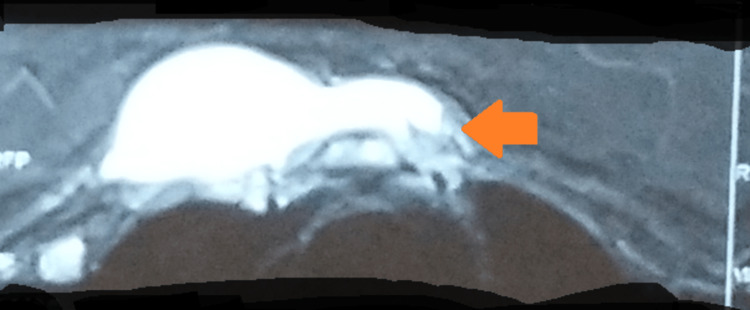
MRI-chest showing a hyperintense, irregular collection anterior to the manubrium sterni (arrow) MRI: magnetic resonance imaging
